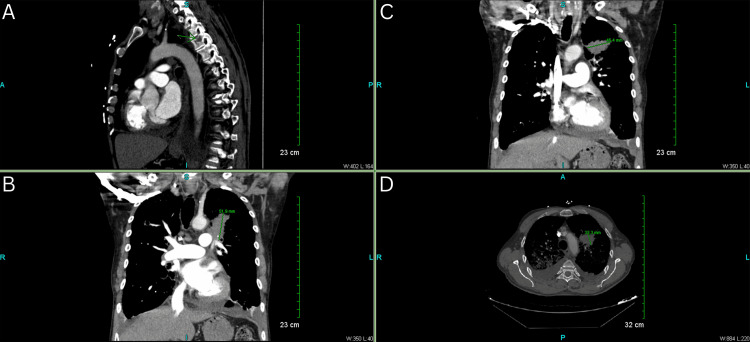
CT chest findings demonstrating lytic lesions involving the right seventh rib, left ninth rib, and T4 vertebral body (A). Additionally, a mass-like opacity with spiculated margins in the left upper lobe perihilar region is observed, measuring 5.2 cm craniocaudally (B), 4.6 cm transversely (C), and 3.3 cm anteroposteriorly (D). Multifocal pulmonary consolidation suggestive of pneumonia and bilateral pleural effusions are also observed. CT: Computed tomography
